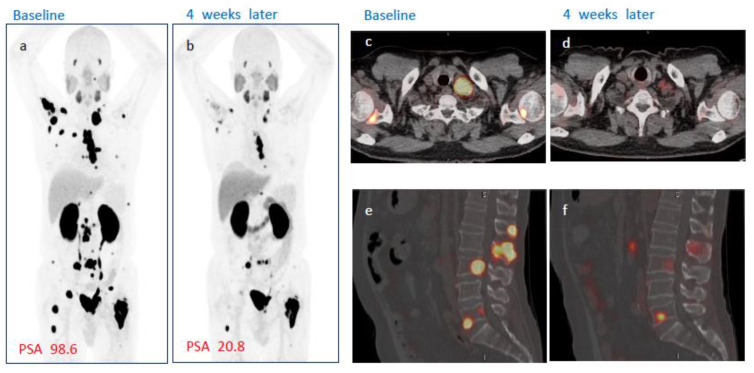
A case of a subject showing response to [^177^Lu]Ludotadipep. (a,c,e) Baseline [^18^F]Florastamin PSMA PET/CT MIP, fused axial and sagittal images. (b,d,e) PSMA PET/CT images four weeks after administration of [^177^Lu]Ludotadipeps (a,b) MIP images show decreased tumor burden in the bone lesions and lymph nodes. (c,d) Axial fused PSMA PET/CT images show marked regression of left supraclavicular lymph node and bone metastases. (e,f) Sagittal PSMA PET/CT images show decreased uptake in the spine lesions.
